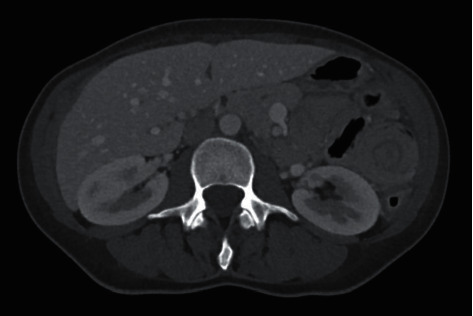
CT scan showing the “Target Sign.”
